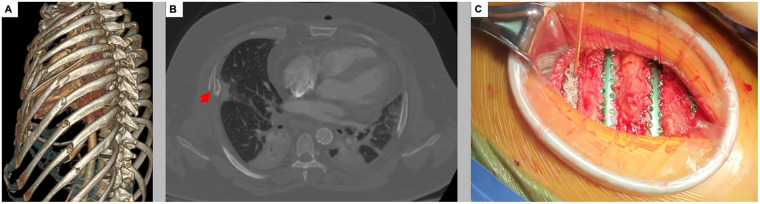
Rib fracture fixation with PEEK and titanium plates. (A) Three-dimensional CT reconstruction demonstrating multiple displaced rib fractures. (B) Axial CT image showing a displaced lateral rib fracture (red arrow). (C) Intraoperative view after surgical stabilization of the chest wall using PEEK and titanium plates.
